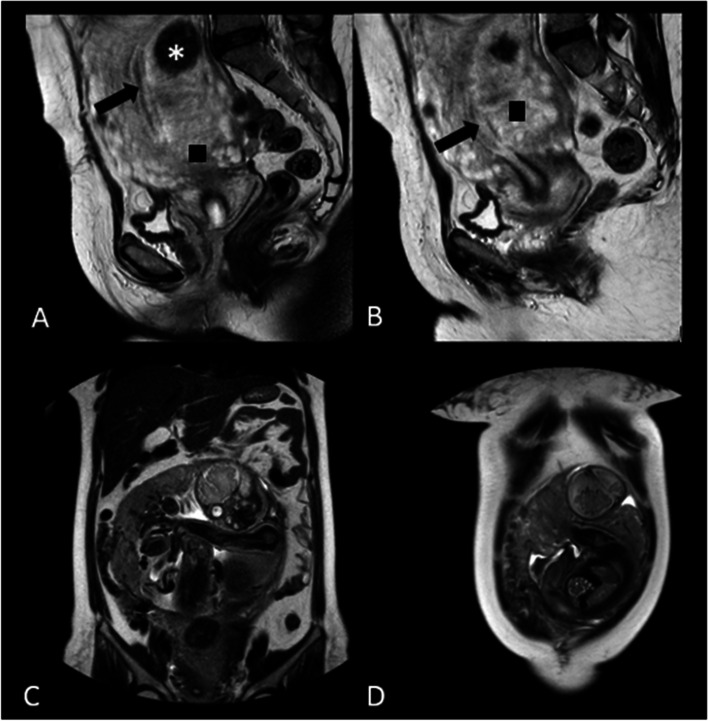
Interstitial pregnancy. Initial interpretation of magnetic resonance images revealed heterogenous hypersignal structure within the lower segment of the uterus that seemed to invade the cervical stroma () which erroneously was diagnosed as lower uterine/cervical mass; however, surgical findings confirmed that this was abnormally adherent invasive placenta of interstitial pregnancy. Retrospective re-evaluations of MRI were as follows: Sagittal (A, B) T2-weighted HASTE MR images showing intramural myoma (*) within the posterior wall of the uterus. Note that the endometrial cavity is completely empty (arrow). Coronal (C, D) T2-weighted HASTE MR images (obtained more cranial than A, B at the level of abdominal cavity) show eccentrically located fetus with overlying thin myometrium. Relationship of pregnancy and utero-tubal junction or the interstitial portion of fallopian tubes was imprecise as a result of the fetus size. The patient underwent successful termination via C-section and the finding at surgery confirmed the interstitial pregnancy
